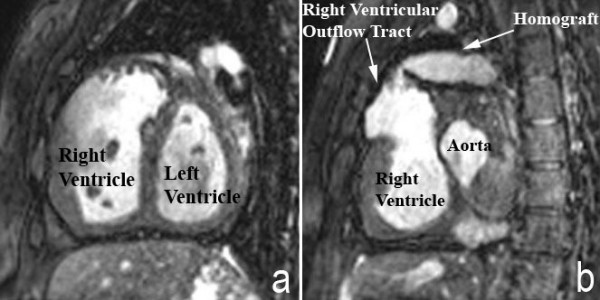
Cardiac magnetic resonance imaging in an adult patient with tetralogy of Fallot repaired in childhood with an infundibular muscle resection and placement of a conduit from the right ventricle to the pulmonary arteries (a) has results in dilation and hypertrophy of the right ventricle. Additional images (b) show the stenotic and tortuous homograft conduit responsible for the dilated and hypertrophied right ventricle.
